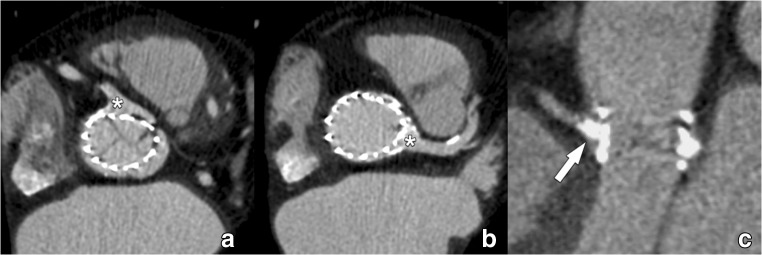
Relation between the coronary ostia and the deployed THV. Both self-expandable and balloon-expandable THVs are designed not to obstruct the coronary ostia, with self-expandable Corevalve and Evolut protheses extending into the ascending aorta by design, leaving the coronary ostia open (a, b). When coronary obstruction occurs, it is not by the THV but secondary to displaced calcified native leaflet remnants that migrate during deployment of the THV in the aortic sinus to the vicinity of the coronary ostia. Nevertheless, while CT can detect these migrated calcifications in or near the coronary ostia (c), the evaluation of luminal patency is less obvious, mostly dependent on local expertise and the quality of the CT scanner used
